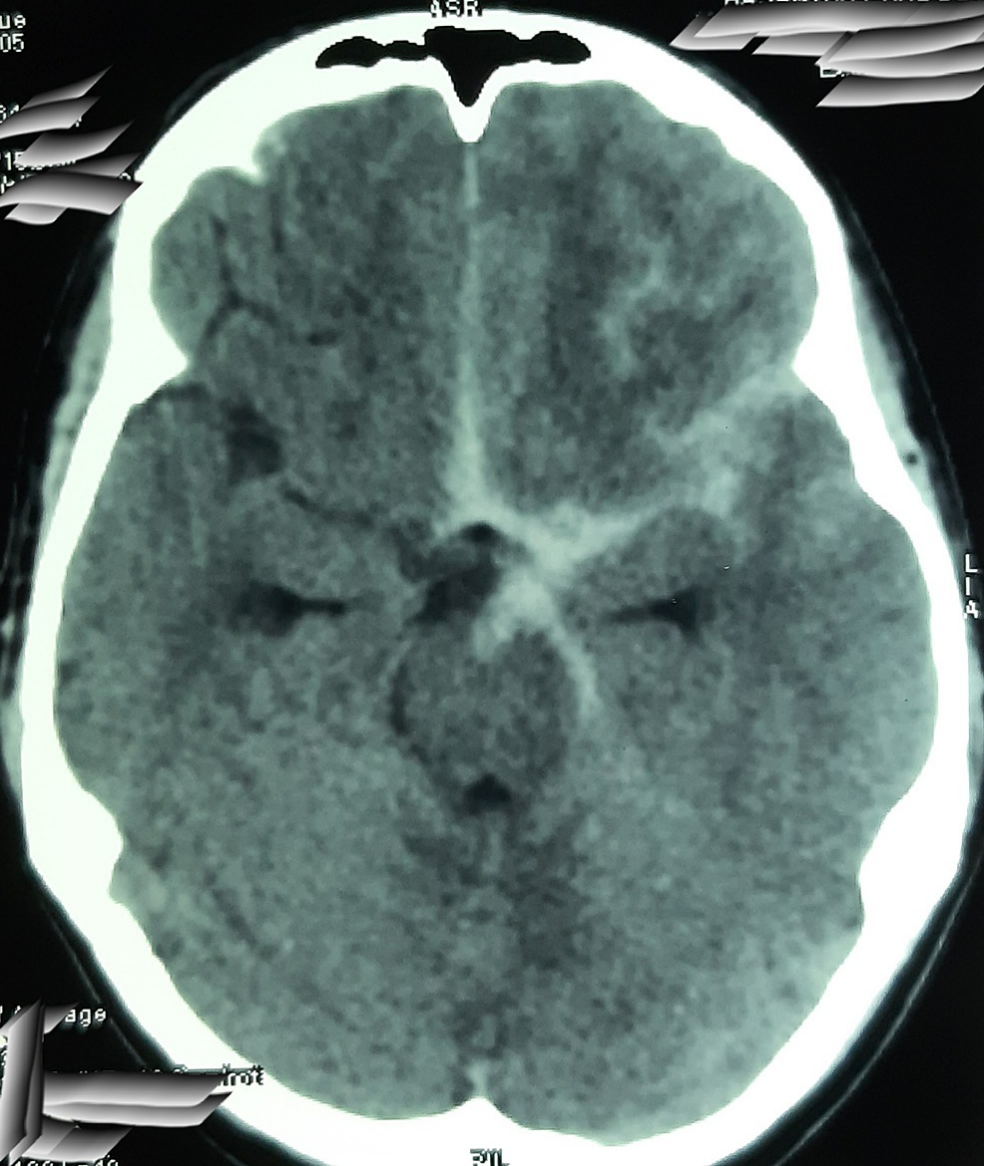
Non-contrast CT brain axial cuts showing subarachnoid hemorrhage
